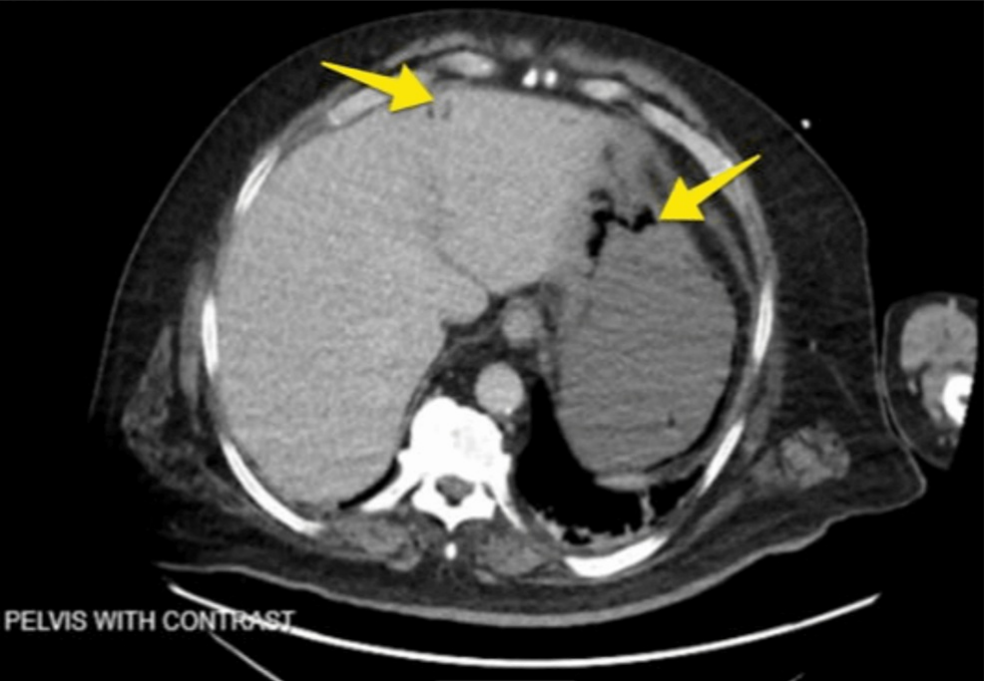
Axial view of CT scan of abdomen and pelvis with contrast showing gastric pneumatosis (right arrow) with portal venous gas (left arrow)
